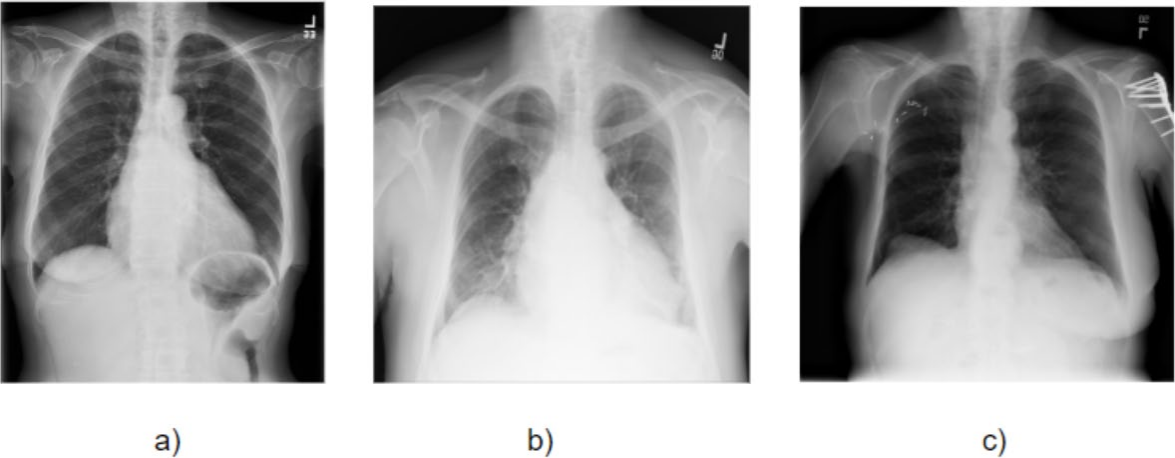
Sample images from the three datasets used in this study: (a) Emory dataset, (b) Chest X-ray Mask dataset, and (c) NIH Chest X-ray dataset. The images demonstrate variations in contrast, patient positioning, and anatomical coverage across datasets
